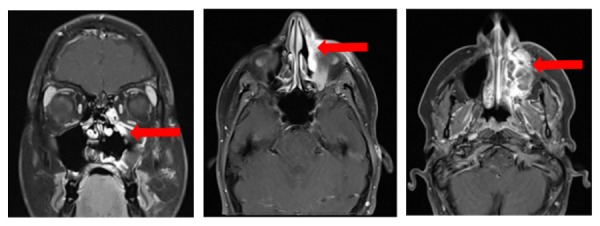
Postsurgical MRI Gadolinium contrast-enhanced T2-weighted coronal and axial MRI images demonstrate a residual T2 hypointense, enhancing soft tissue mass involving the anterior left maxillary sinus, premaxillary soft tissues, and extending​
